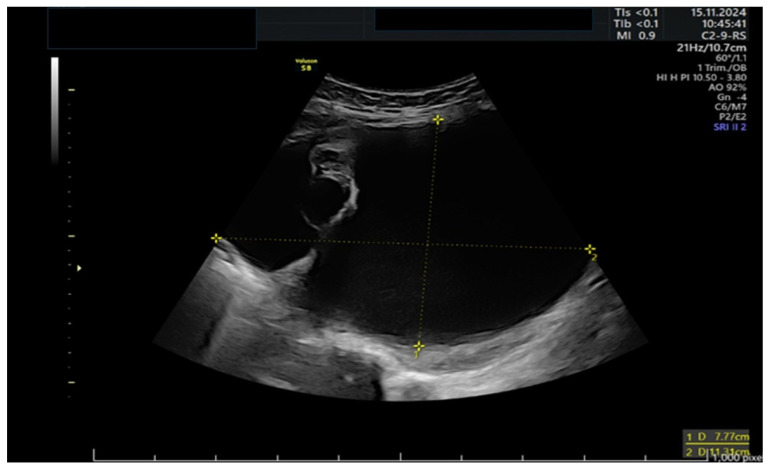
Ultrasound of a large 12 cm tricameral adnexal mass without Doppler signal.
